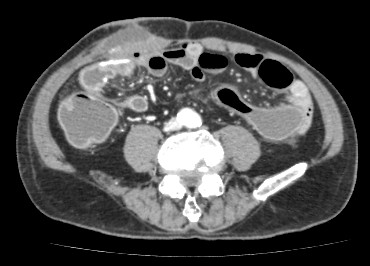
Abdominal computed tomography on the third postoperative day. A CT scan demonstrated that subcutaneous fluid had collected adjacent to the surgical wound and that the colon had become slightly distended and showed no wall thickening.
